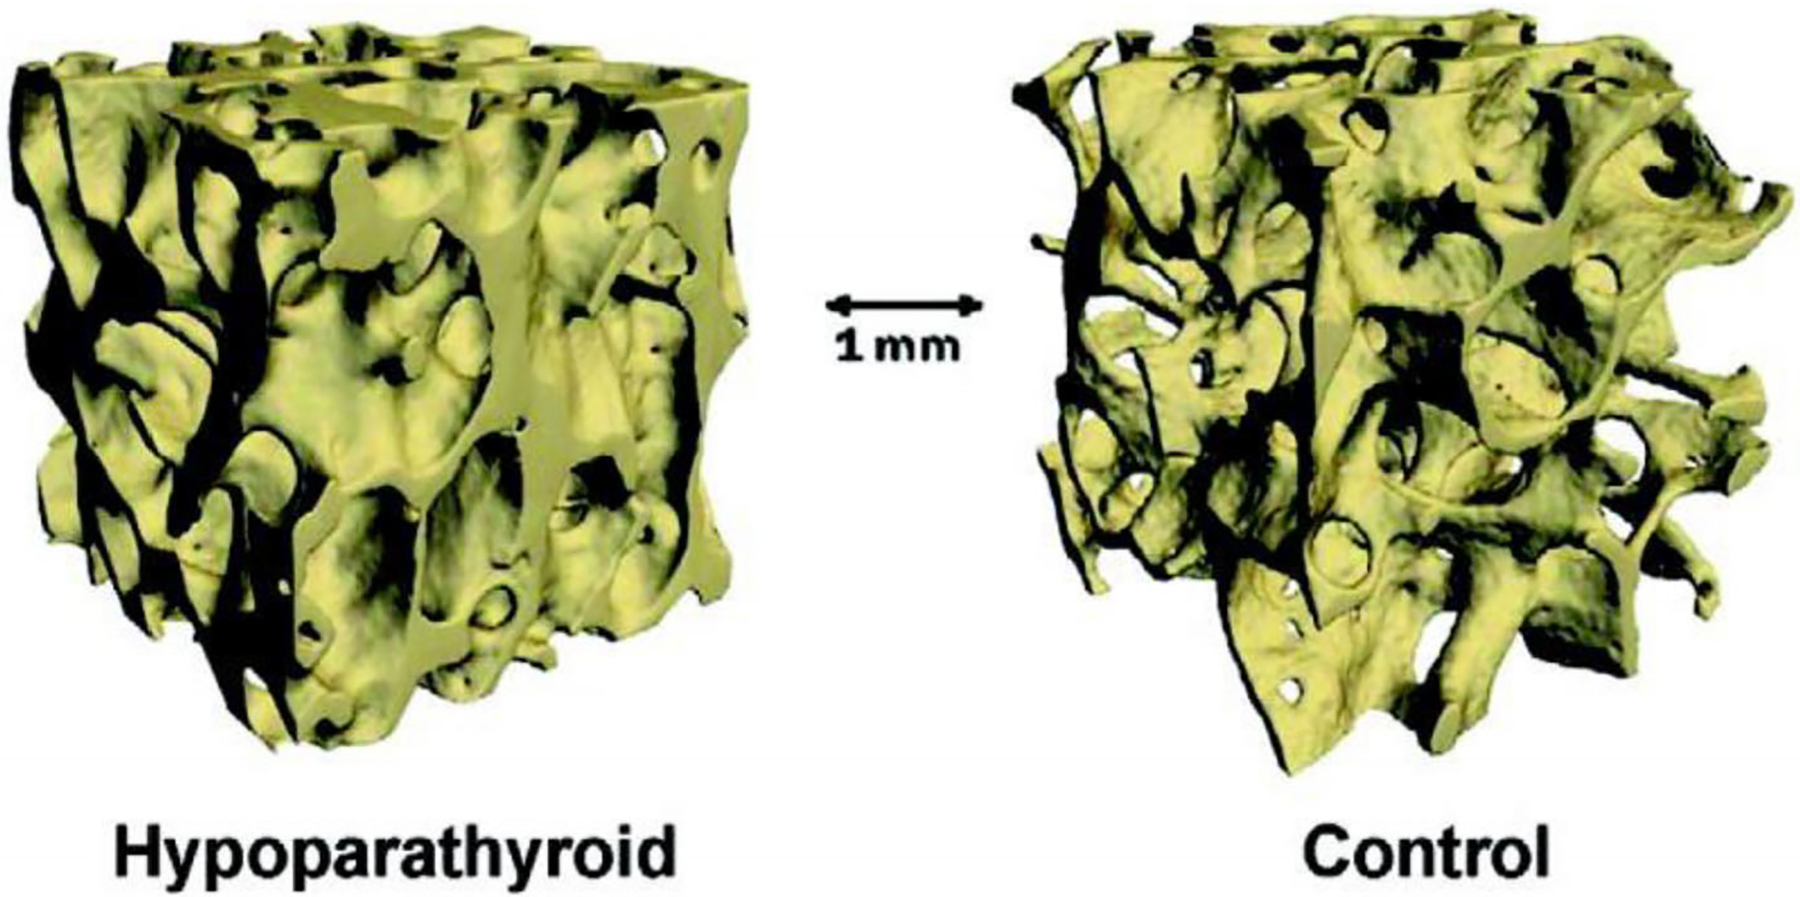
Microcomputed tomographic images of cancellous bone from a hypoparathyroid subject (left) and a control subject (right). Note the higher cancellous bone volume and dense trabecular structure in hypoparathyroidism. Reproduced with permission from Dempster.^(137)^
